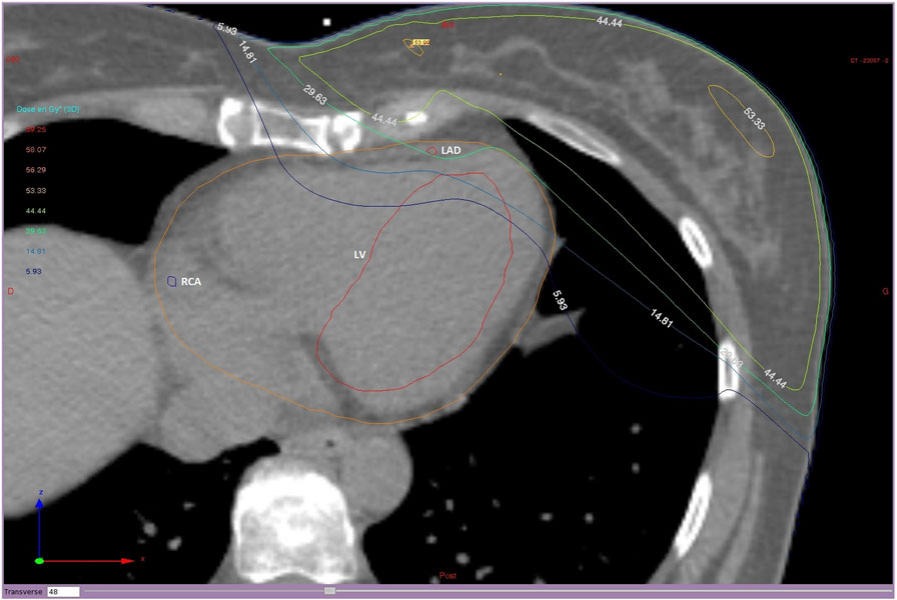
CT dose-planned left tangential breast irradiation showing isodoses and delineated structures: Heart (orange circle), left ventricle (LV), Left anterior descending coronary artery (LAD) and right coronary artery (RCA)
